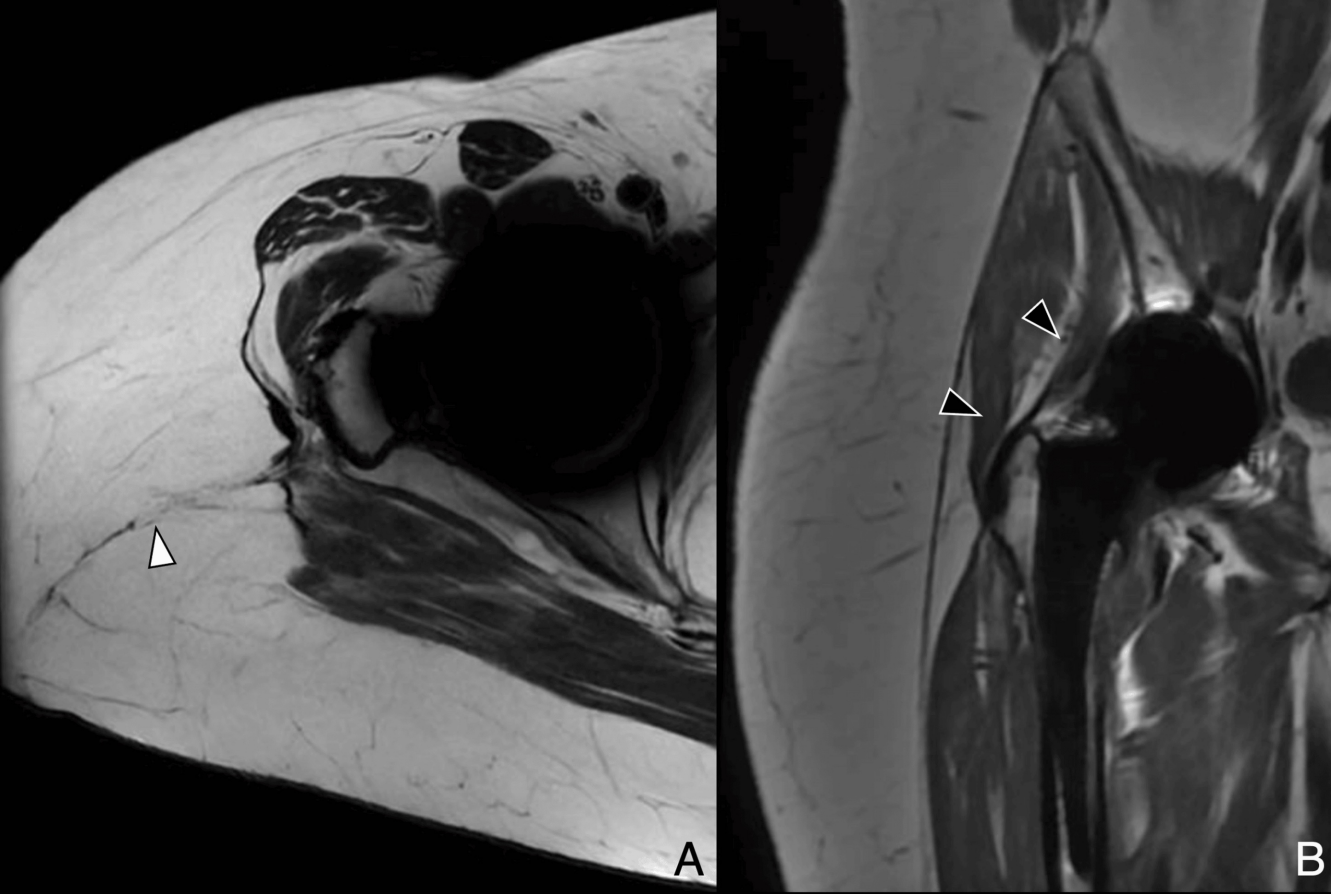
Posterior approach: postoperative MRI (A) Axial T2-weighted image showing hypointense scar in the subcutaneous tissue (white arrowhead). (B) Coronal MAVRIC (multi-acquisition variable resonance image combination) sequence demonstrates preserved abductor tendons (black arrowheads). Image Credit: Juan Pablo Muñoz, corresponding author
